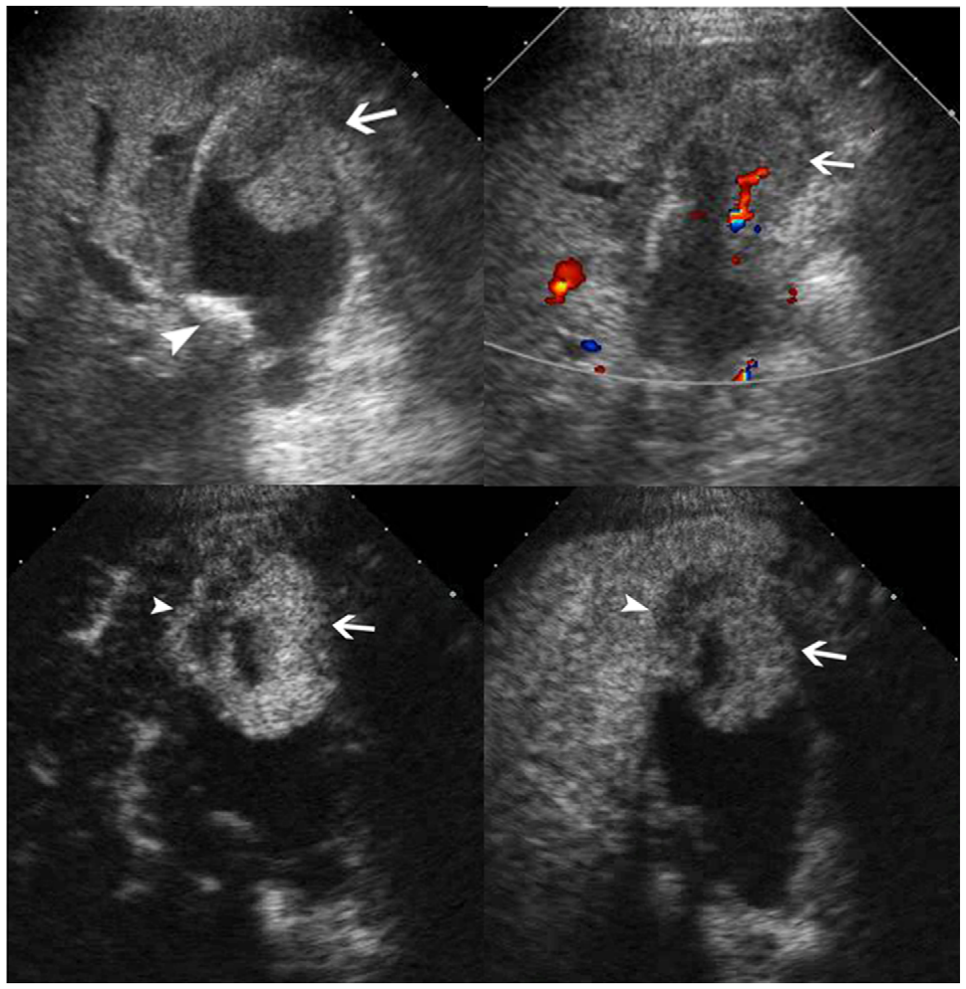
Adenocarcinoma in gallbladder. Upper left, conventional ultrasound shows an isoechoic mass (arrow) and a stone (arrowhead) in the gallbladder; Upper right, color flow imaging shows intralesional vascularity (arrow); Lower left, the lesion (arrow) shows hyper-enhancement during the arterial phase and the infiltration (arrowhead) to the adjacent liver is seen; Lower right, the lesion (arrow) shows hypo-enhancement during the venous phase and the infiltration (arrowhead) to the adjacent liver is seen.
